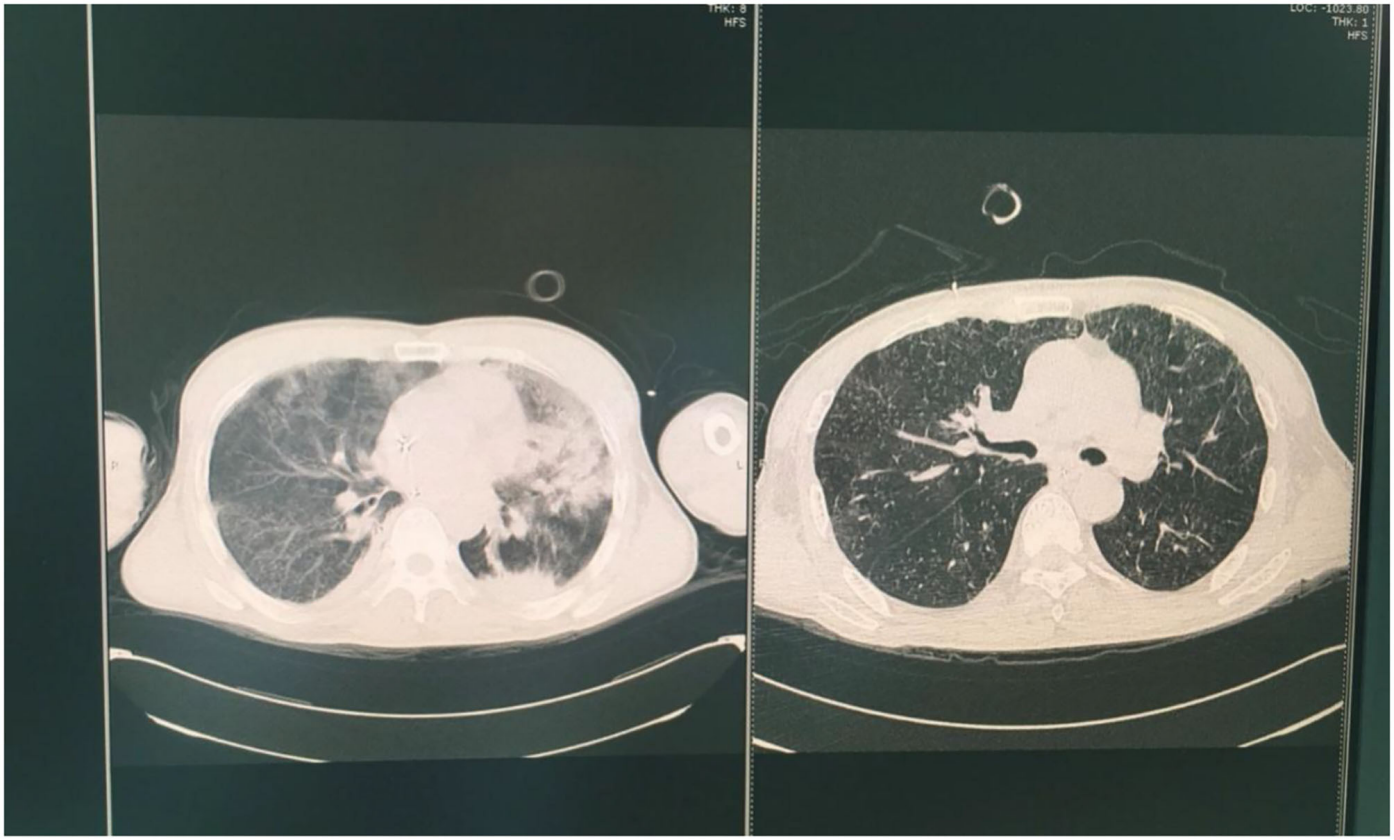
The chest CT images on the second (left) and seventh day (right) after weaning.
